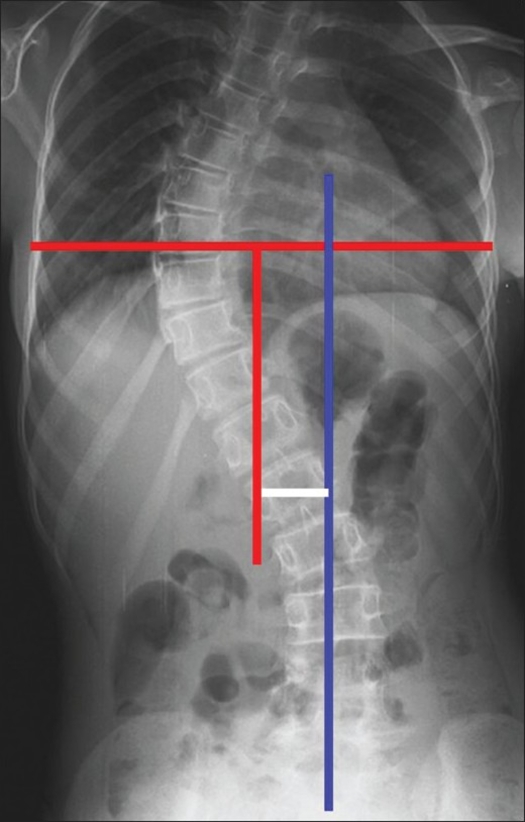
Measurement of trunk balance: X-ray of dorsal, lumbar spine including lumbosacral junction (anteroposterior view) showing a central sacral line (blue), vertical line (red) bisecting a line drawn from the peripheral edges of the ribs of the apical vertebra. The horizontal distance between the vertical lines (white) quantify the trunk imbalance
